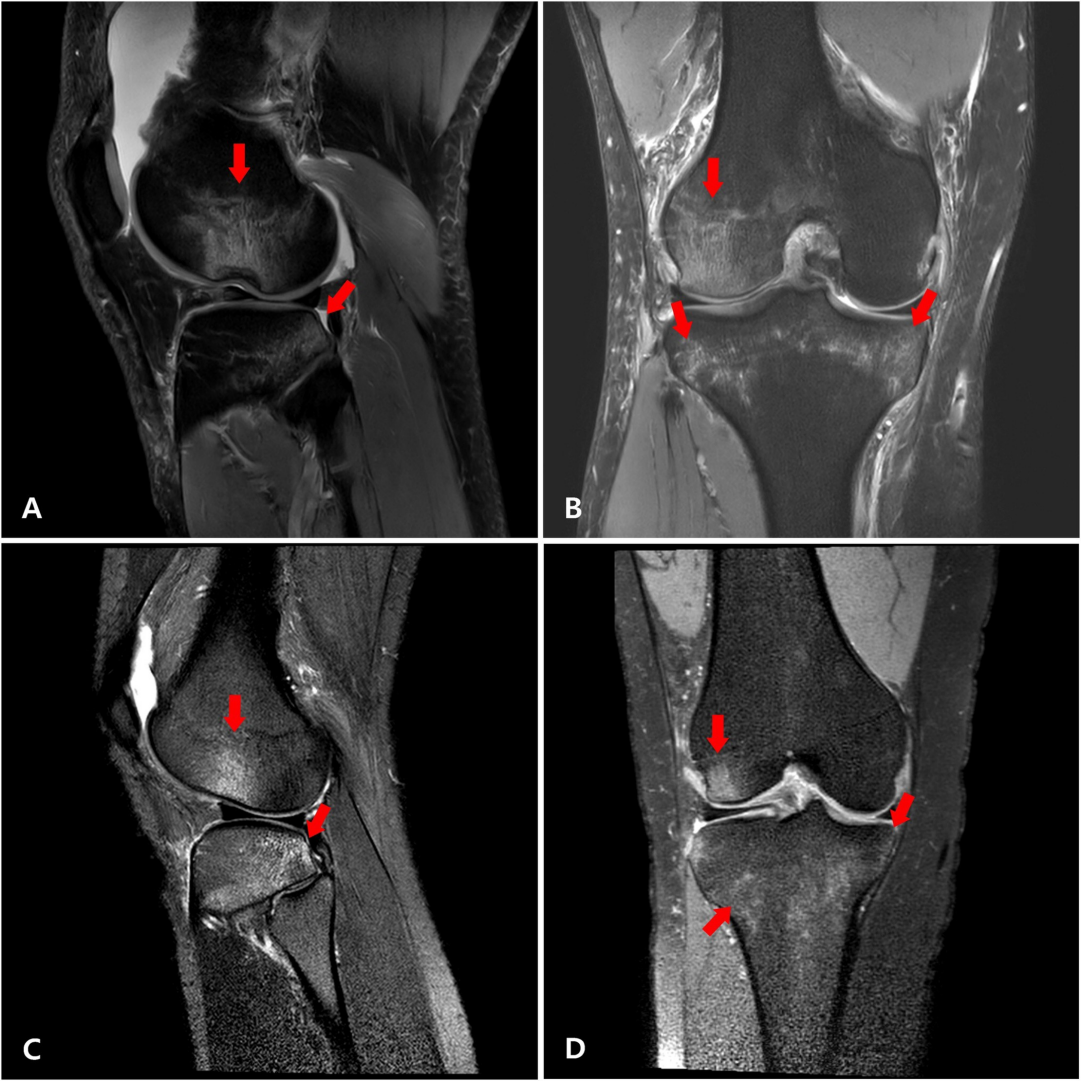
T2-weighted MRI of typical patterns of osseous lesions in acute non-contact ACL ruptures. Sagittal (A) and coronal (B) images of a male patient show osseous lesions in the central aspect of the distal lateral femoral condyle and the posterior aspect of the lateral and medial tibial plateau. Sagittal (C) and coronal (D) images of a female patient show the same pattern of osseous lesions.
